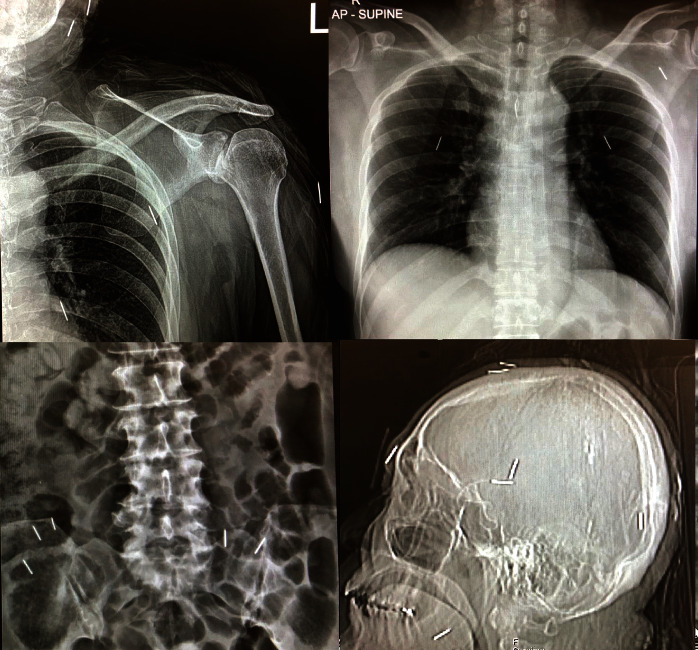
Plain X-ray exhibiting many 1 cm pin-like metallic opacities at the posterior chest wall, paraspinal area of the lumbosacral spines, and skull.
